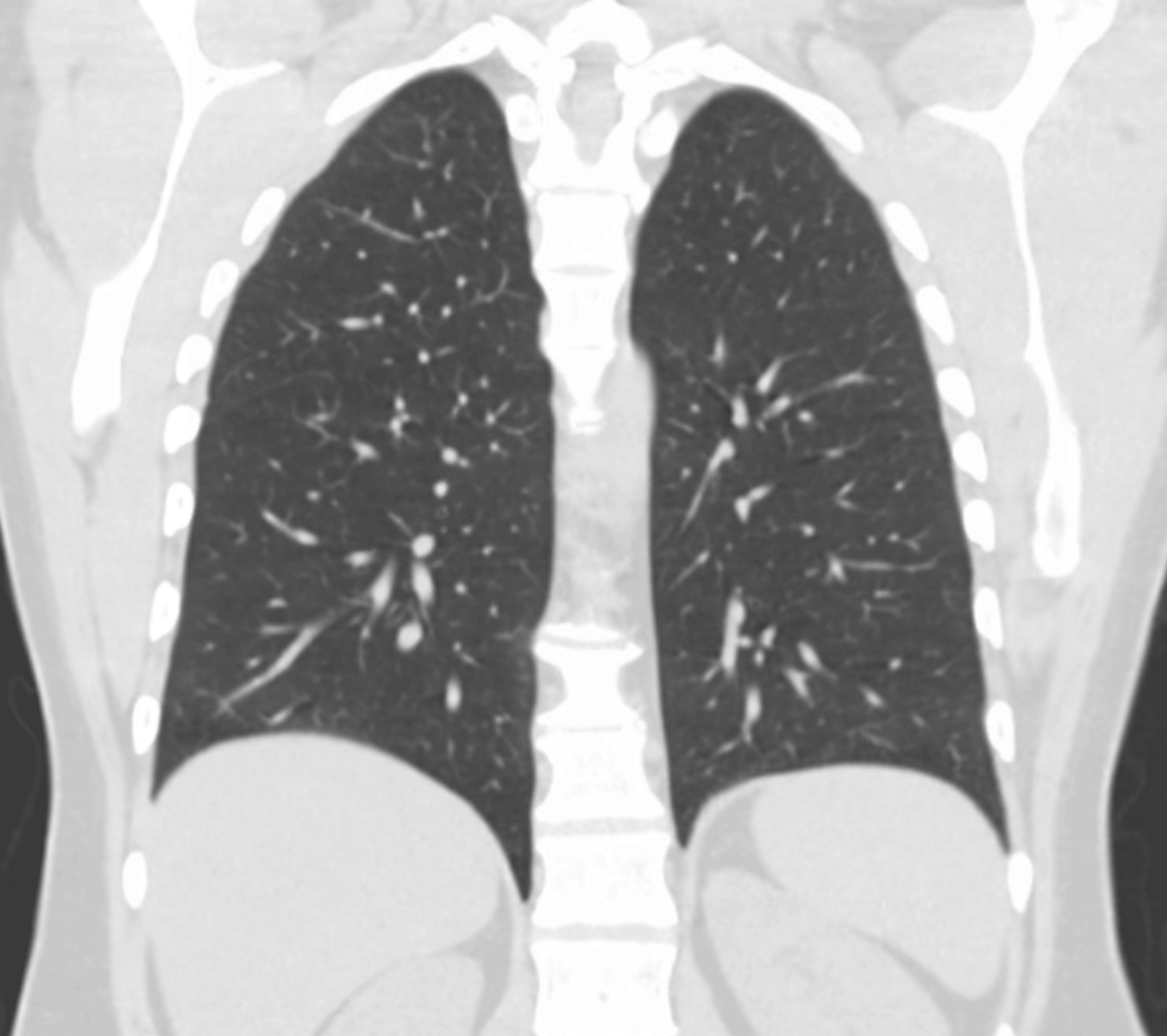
Coronal view of CT chest showing no signs of metastatic disease.
